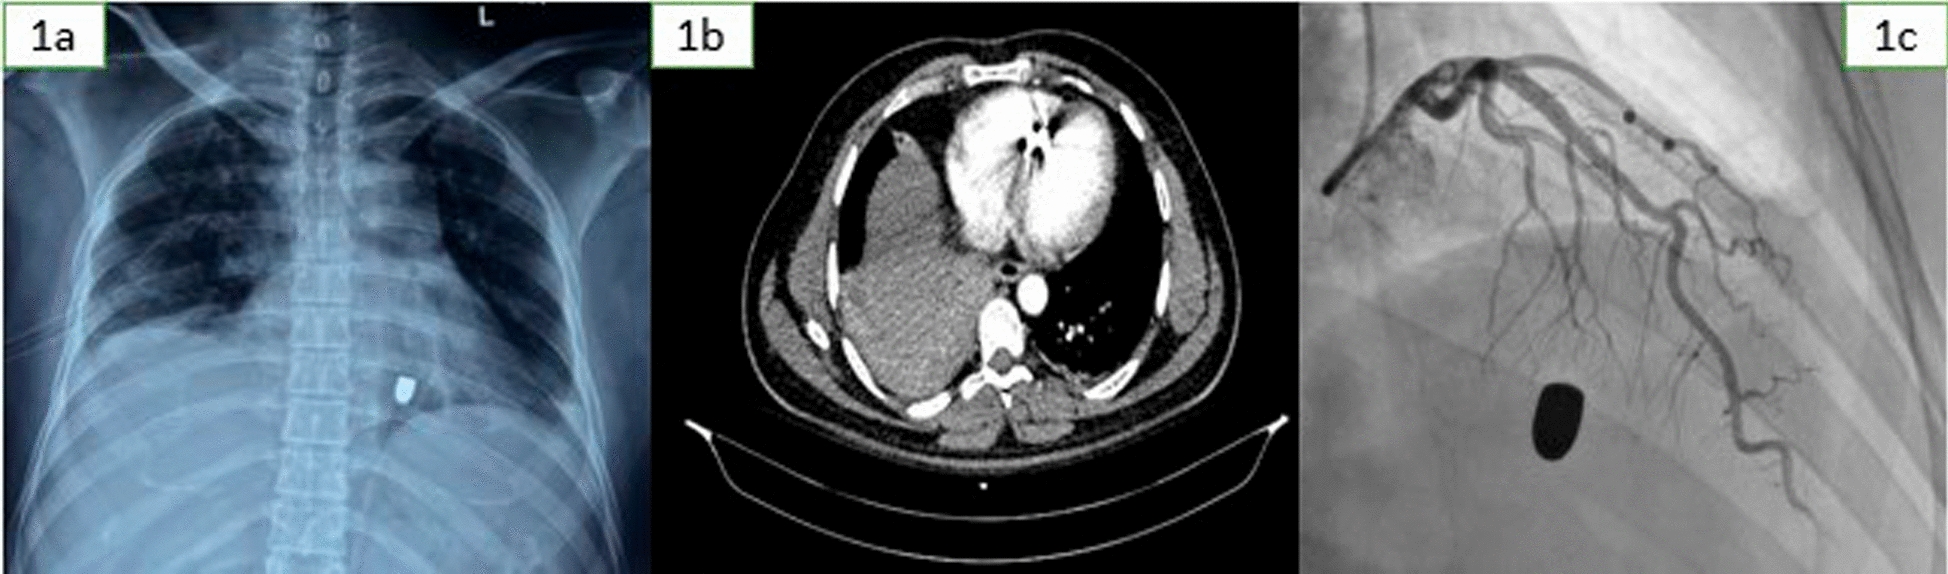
a Chest X-ray posteroanterior view showing the bullet in the mediastinum and intercostal chest drain in the right hemithorax. b Contrast-enhanced computed tomography of the thorax showing bullet in the interventricular septum. c Angiography showing bullet inside the heart with no injury to the coronaries
